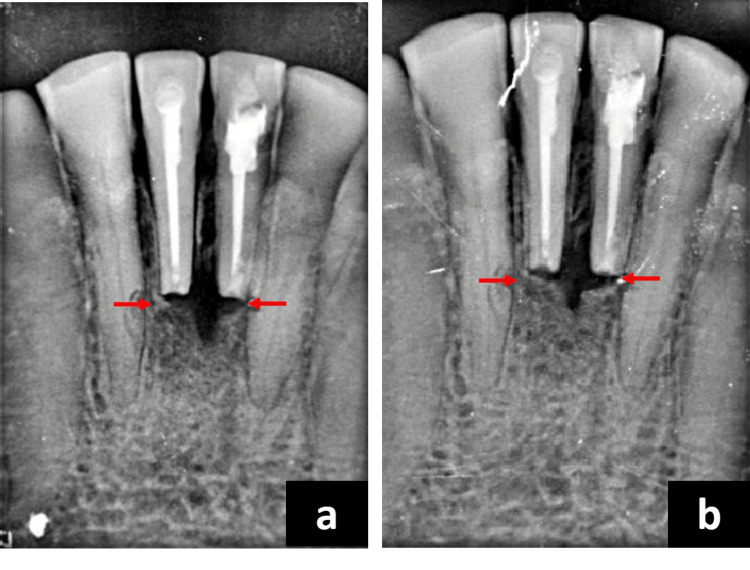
Follow-up radiographs (a) Follow-up IOPA radiograph at six months. (b) Follow-up IOPA radiograph at 12 months shows the progression of healing and bone-fill at the periapical region of 31 and 42. IOPA: intra-oral periapical
